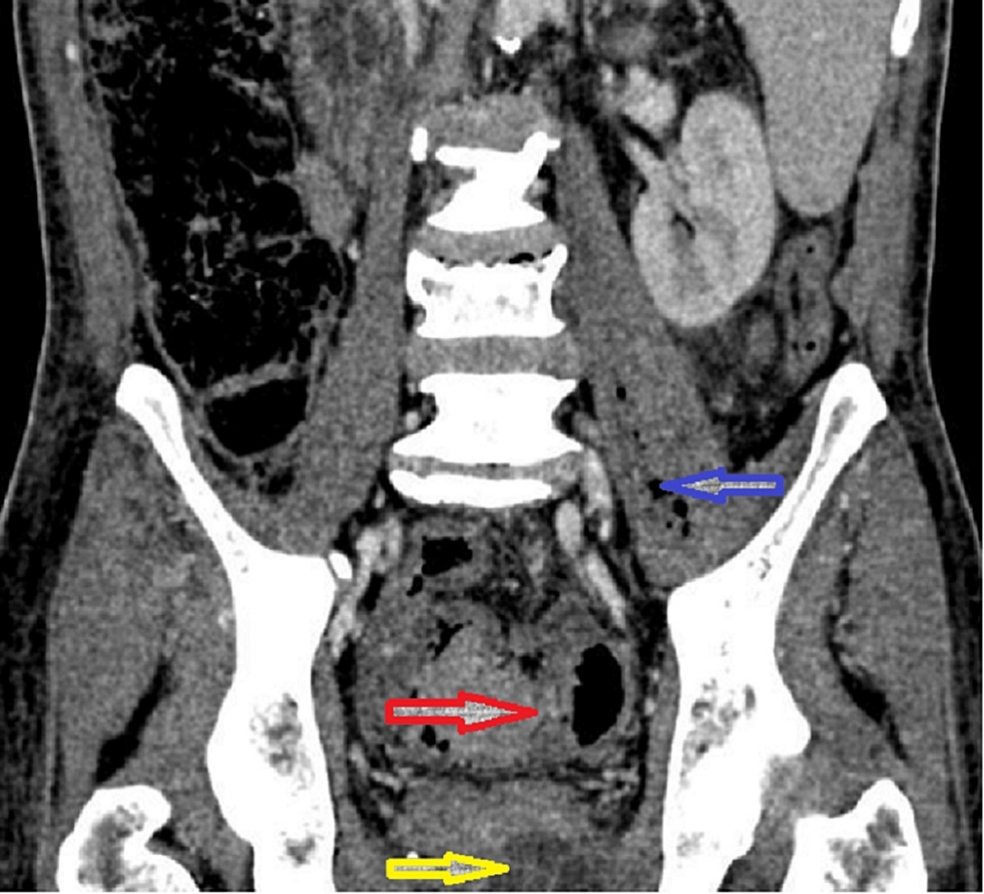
Coronal contrast-enhanced CT image Anterior coronal image of the same CT scan shows a sigmoid mass (red arrow), part of the prostate abscess (yellow arrow), and a left iliopsoas muscle abscess containing air (blue arrow).
